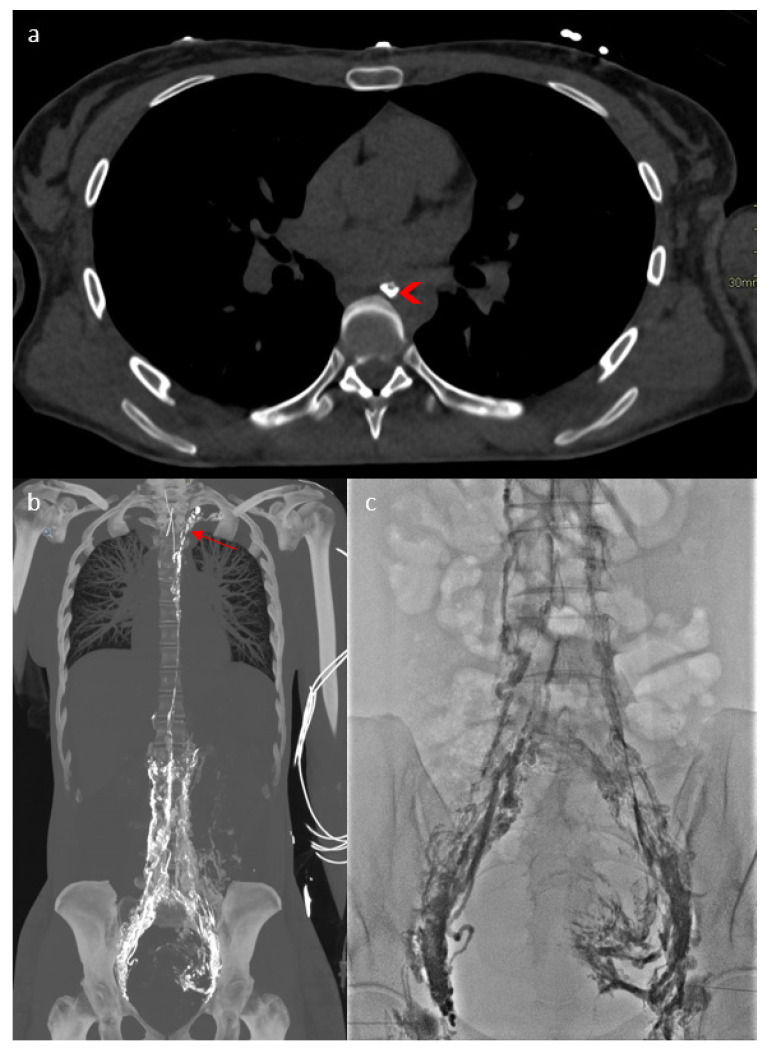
Four-dimensional CT lymphography showing the thoracic duct ((a)—arrowhead), diffuse involvement of the lymphatic network (b) with a “Medusa head” (arrow) aspect of the thoracic duct and lipiodol reflux to the pelvis (c).
